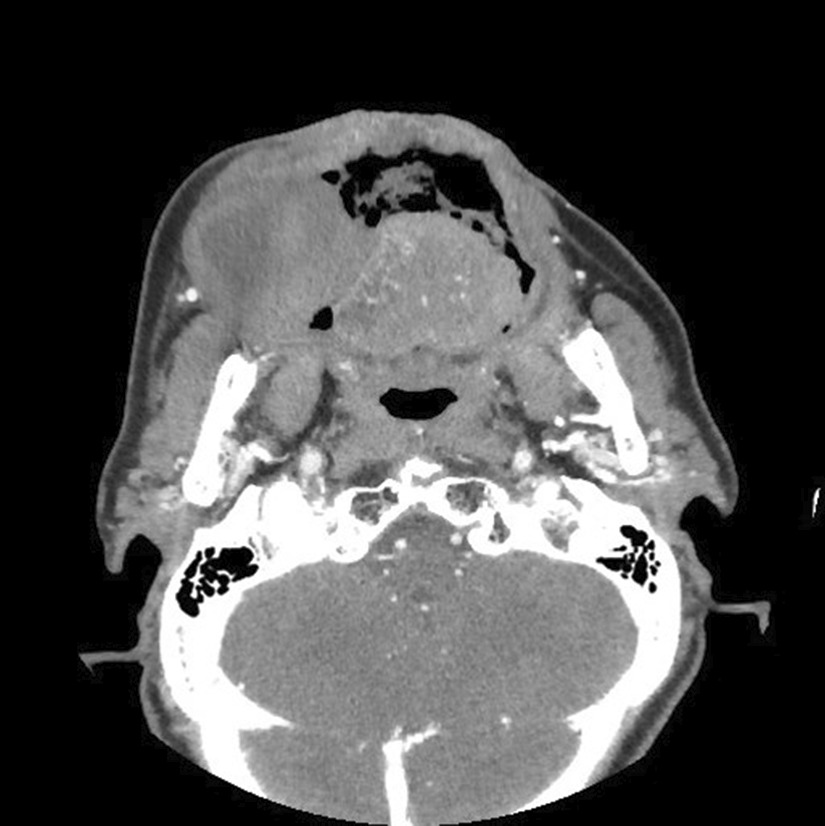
Computed tomography angiogram of the hematomas showing a large hematomas in the right buccal mucosa, with no evidence of arteriovenous malformation
